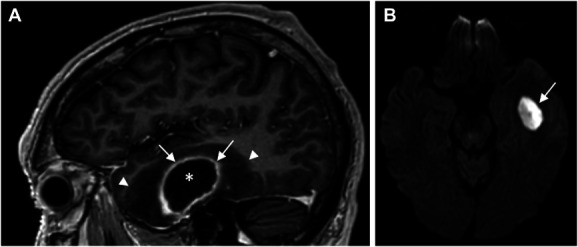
Brain abscess with capsule and surrounding edema. A, T1-weighted MRI after intravenous gadolinium contrast, showing an abscess in the left temporal lobe, with the abscess capsule (arrows) surrounding the abscess cavity (asterisk). Vasogenic edema surrounds the abscess (arrowheads). B, Diffusion-weighted MRI in the horizontal plane, showing highly restricted diffusion of the content of the abscess cavity (arrow), meaning that the content is highly viscous, ie, pus.
